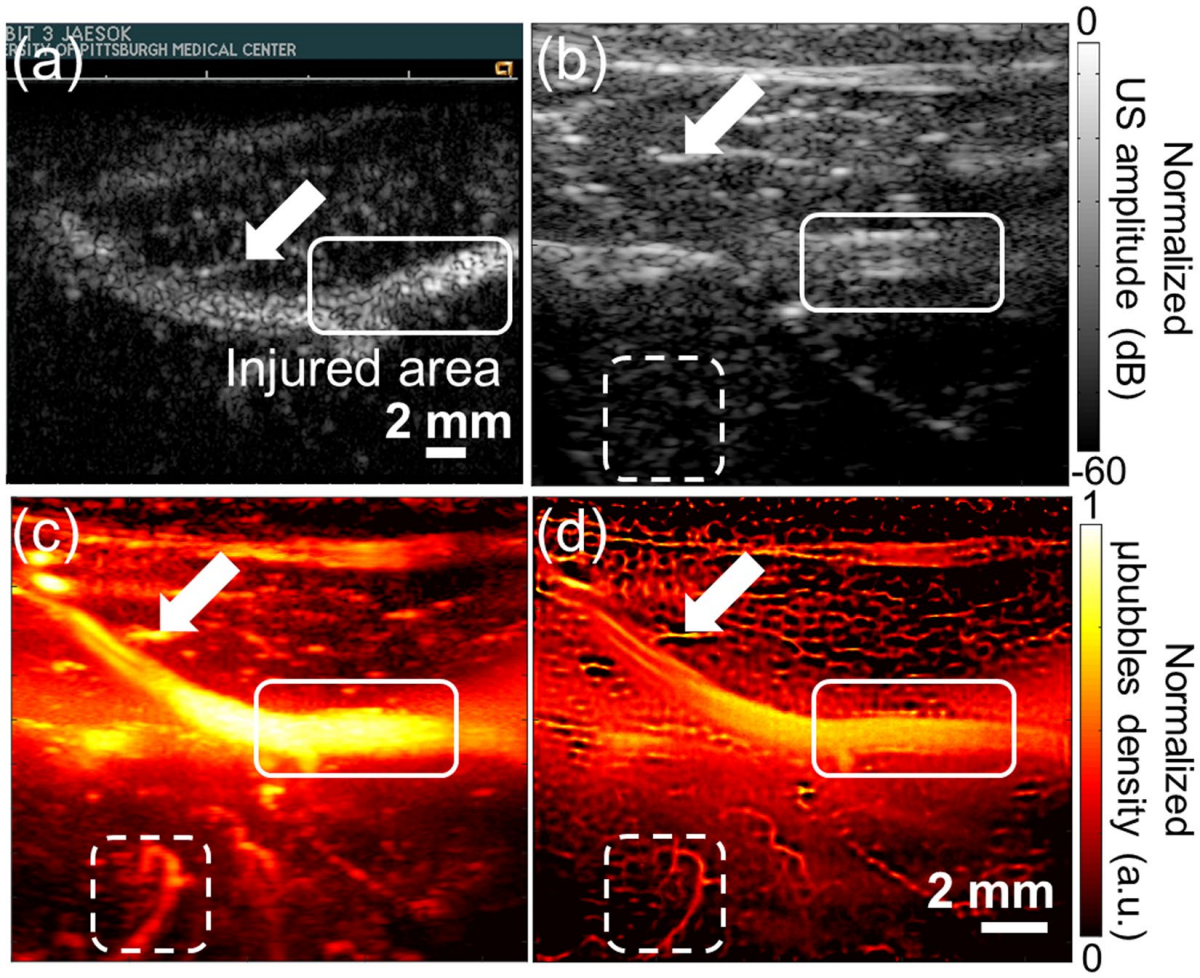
Comparison of several imaging modalities. (a) Cadence^TR^ contrast-enhanced imaging with microbubbles acquired by commercial ultrasound scanner (Sequoia 512, Siemens), (b) conventional B-mode imaging, (b) temporal MIP vascular imaging, (d) proposed super-resolution imaging of ROI. Same raw data is used to reconstruct images (b–d). The white solid rectangle represents balloon-injured area that the plaque is expected to be developed. The white arrow indicates the same vessel branch that shows a correlation of images acquired by two different ultrasound scanners. White dashed rectangle represents selected ROI used in Figs 2 and 3.
